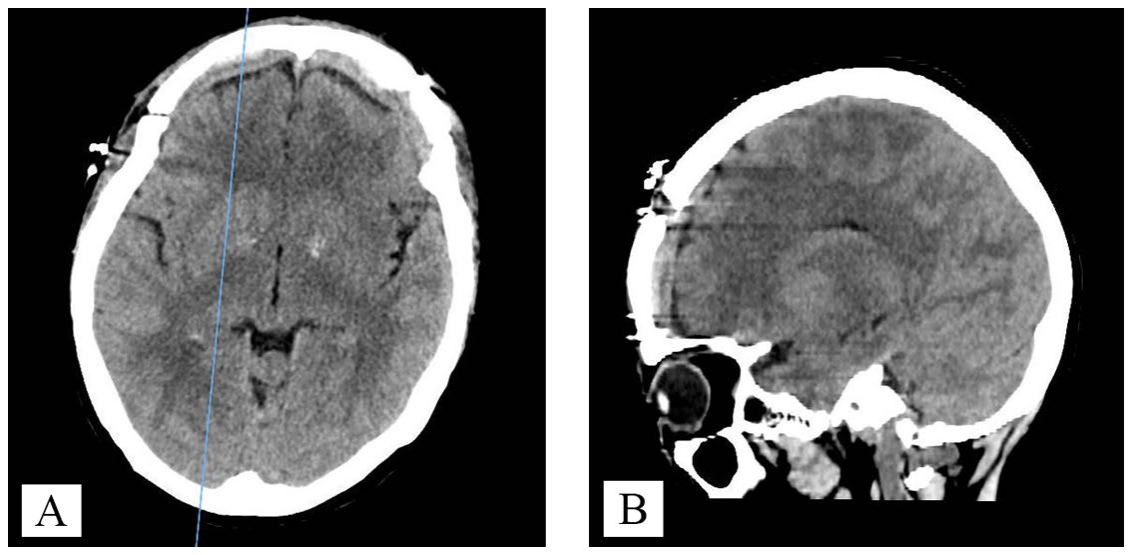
Postoperatively, a cranial CT scan was performed: (A) cross-section, (B) sagittal section.
